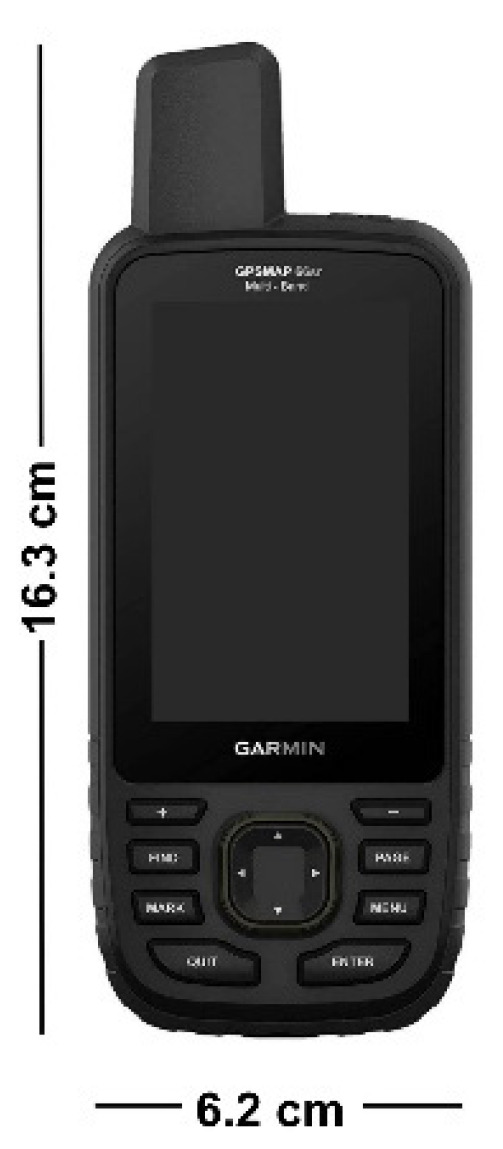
Garmin GPSMAP 66sr with its quadrifilar helix antenna at the top left.
